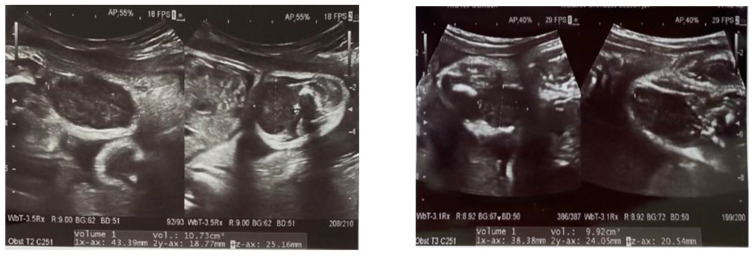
Prenatal ultrasound images at 30 weeks of gestation, showing an oval mass, hypoechoic with calcification on the thigh, with no evidence of bone involvement.
